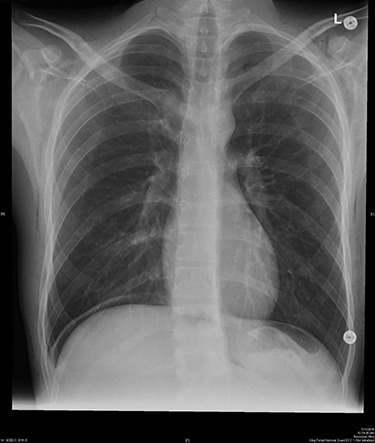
Final pre-discharged x-ray showed improvement of the SE compared to the intra-operative x-ray.
